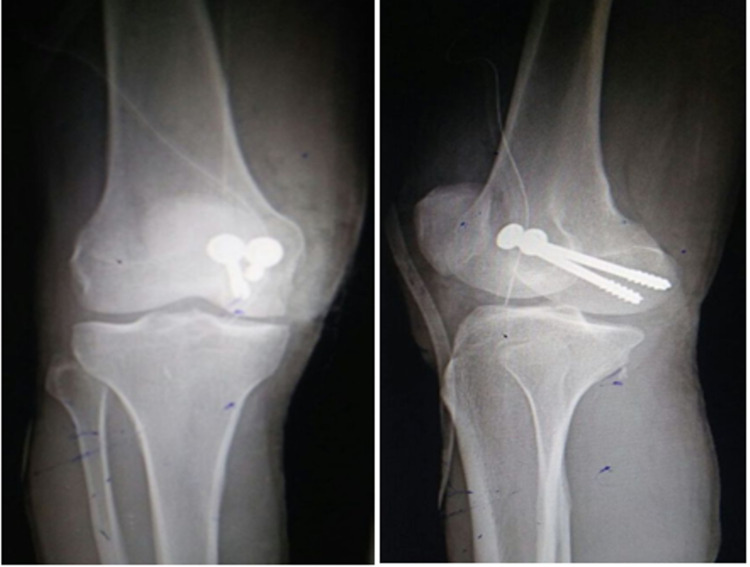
Anteroposterior and lateral radiograph of medial condyle Hoffa's fracture after fixation.
